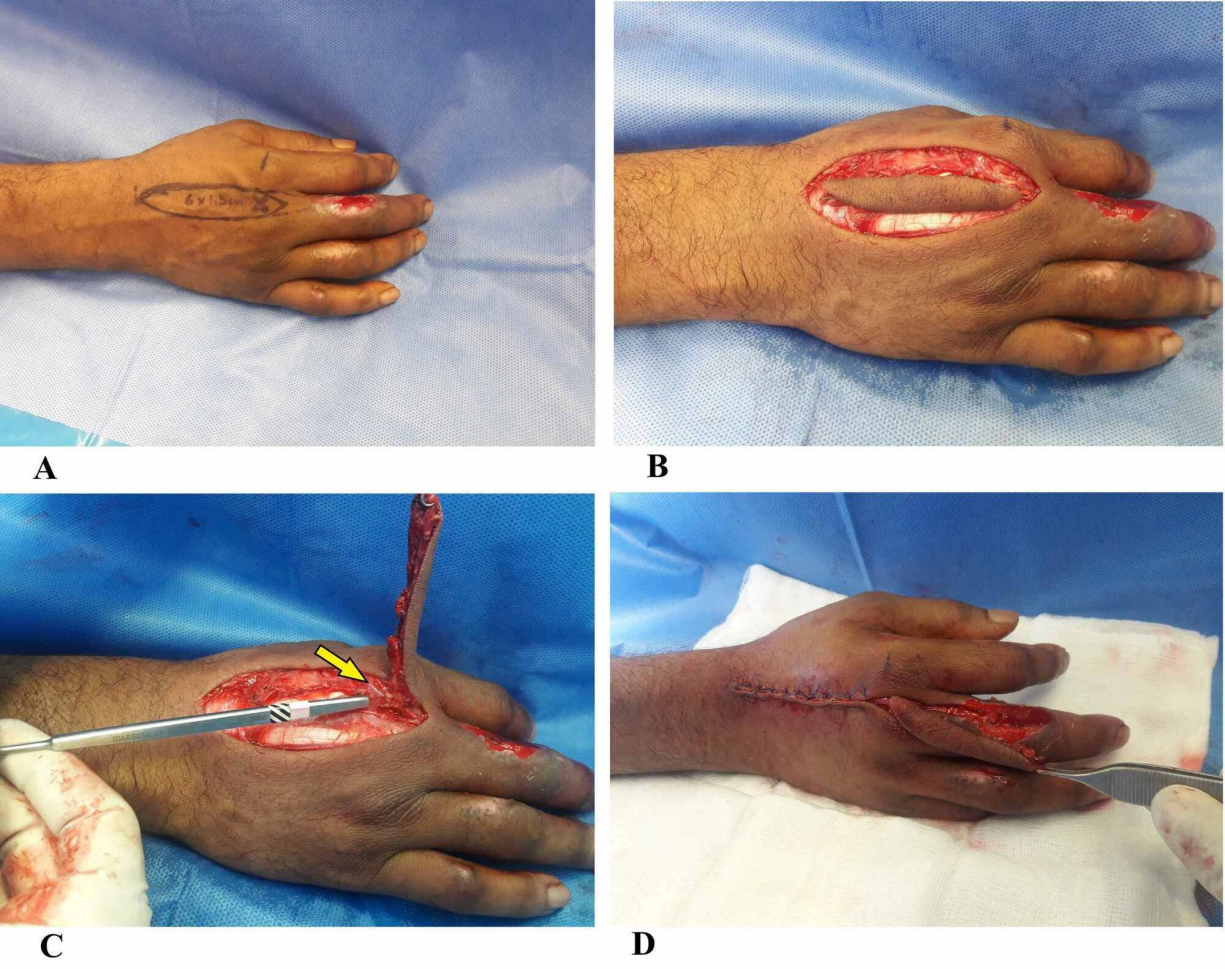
Intra-operative images (A) Intra-operative image of DMCAP flap marking with Doppler perforator. (B) DMCAP flap is islanded. (C) DMCAP cutaneous perforator at the level proximal to second metacarpal head (yellow arrow). (4) The flap is islanded fully and rotated by 90 degrees to reach the defect DMCAP: dorsal metacarpal artery perforator
